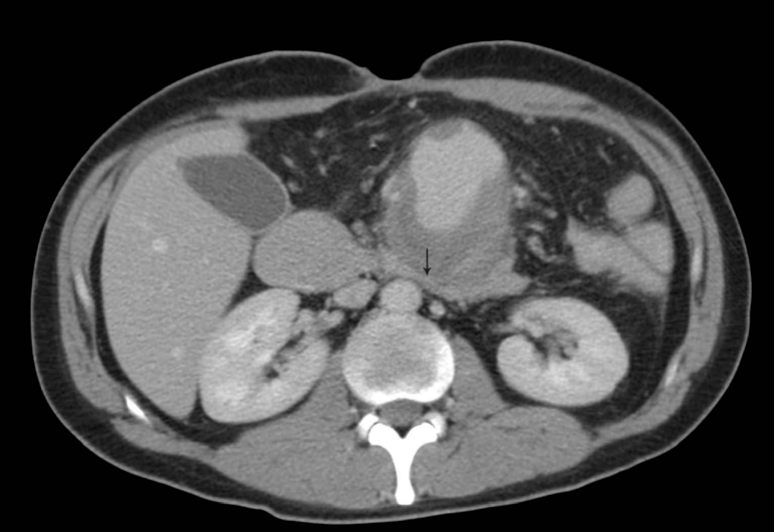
Axial CT images demonstrating the SMA pseudoaneurysm compressing the fourth part of the duodenum (arrow).
